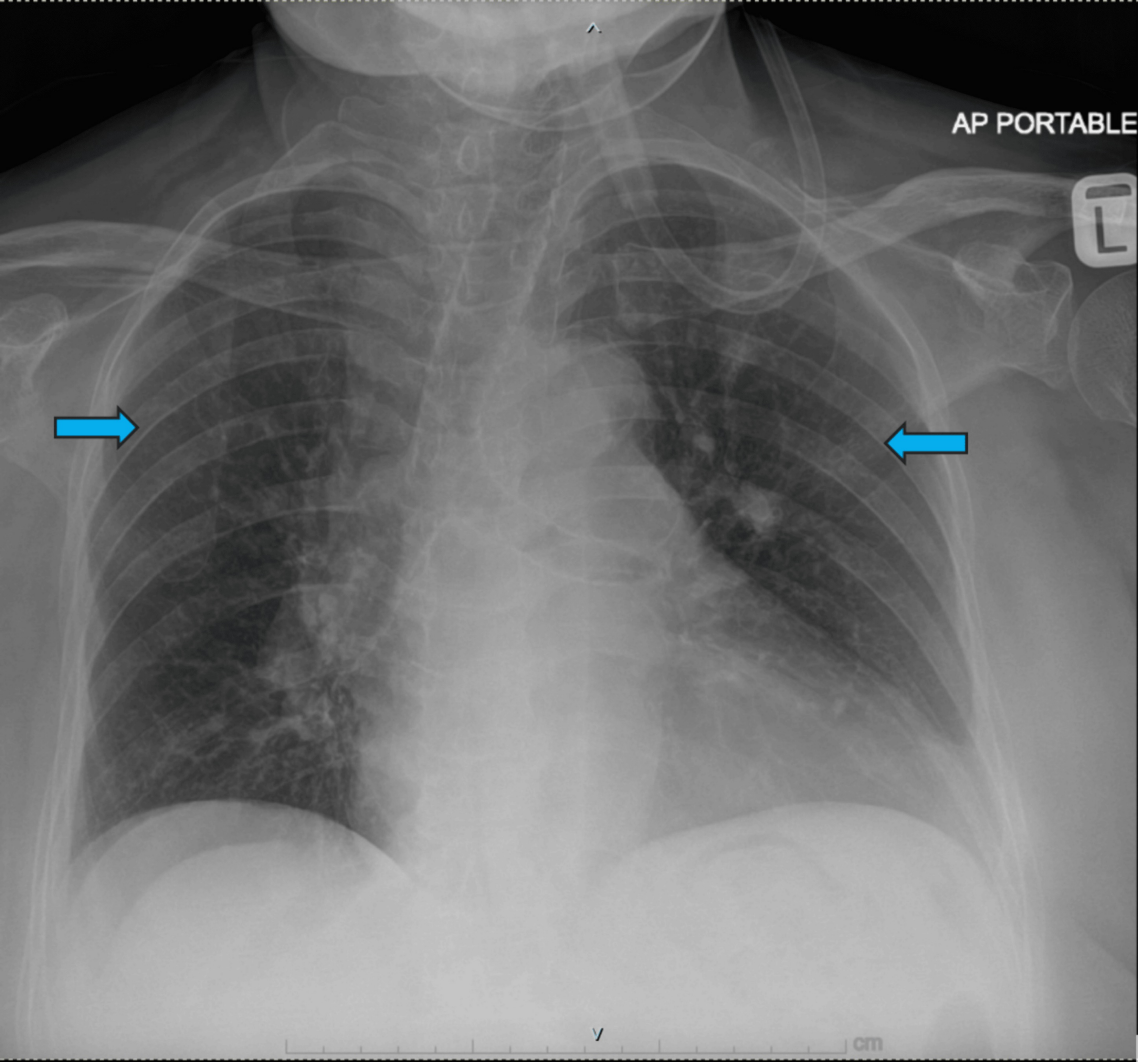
Chest X-ray showed no signs of pneumonia.
